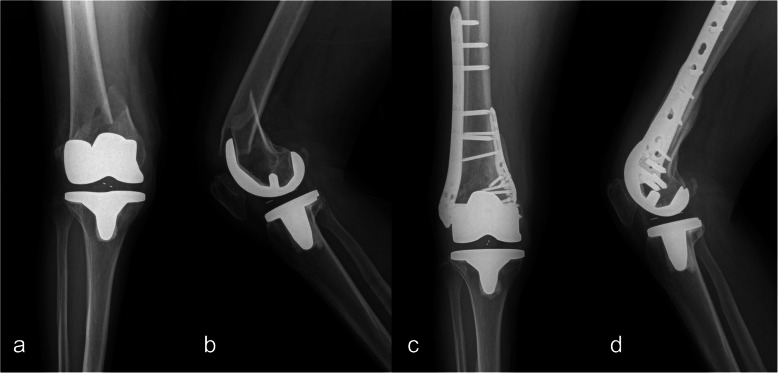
Distal femoral periprosthetic fracture after slip-down in a 68-year-old female patient. a Preoperative knee AP and b lateral radiograph show Su classification type 2 in periprosthetic fracture of the distal femur. Minimally invasive plate osteosynthesis with dual LCP was performed. Post-operative 1-year knee ROM was 125° and JLETS score was 42. c Post-operative knee AP and d lateral radiograph at 1 year show well-united fracture and satisfactory alignment
